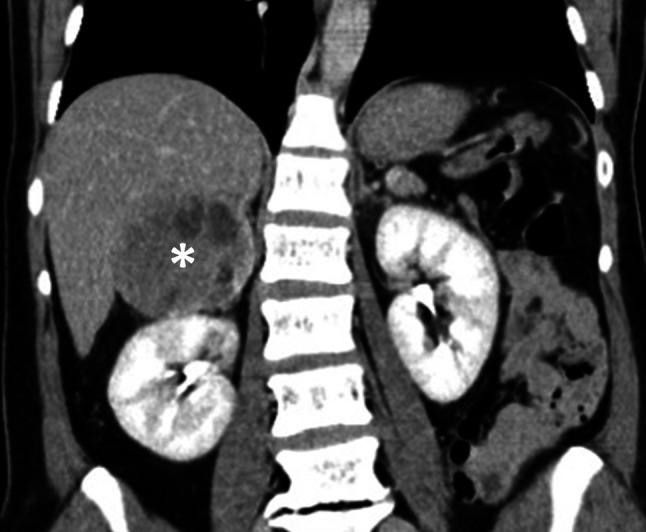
Computed tomography coronal view confirming presence of right adrenal mass (denoted with *asterisk*)
